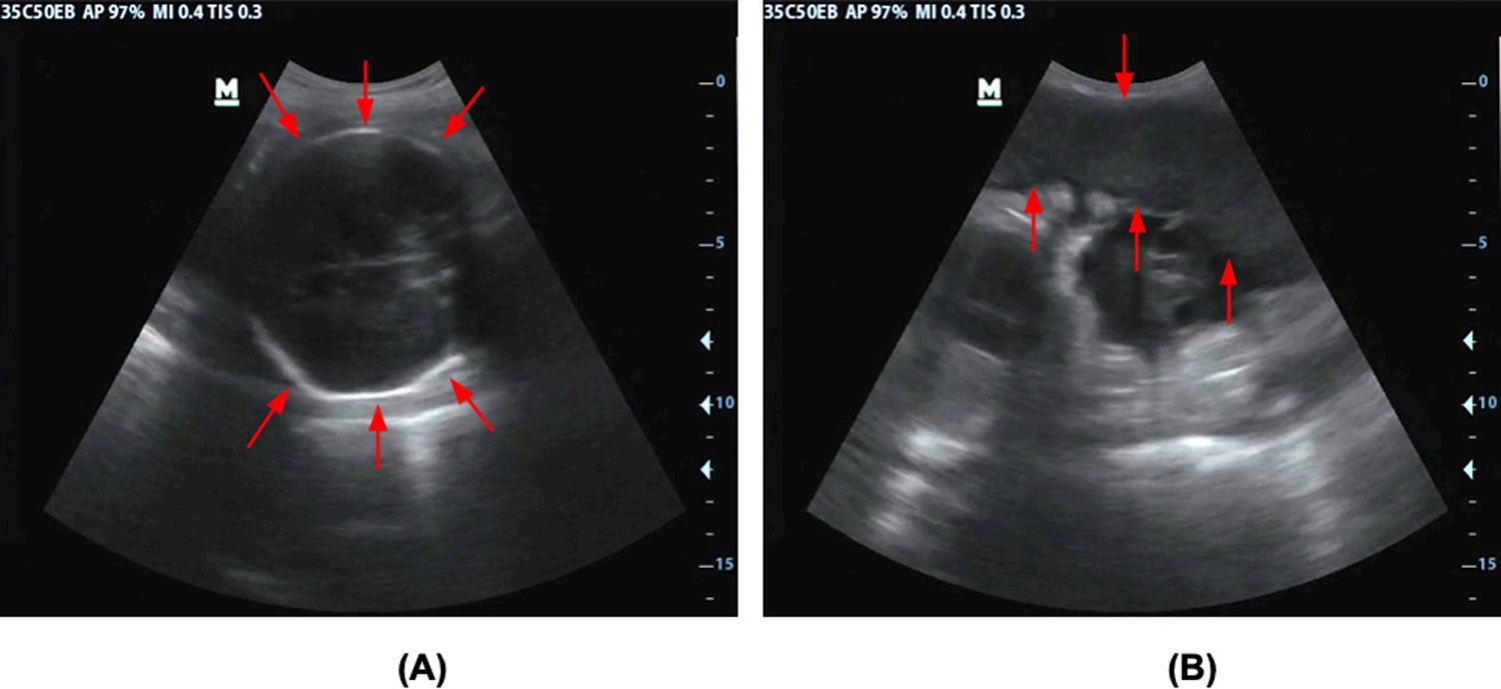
Representative 2D ultrasound images from VSI acquisition. Red arrows identify the head (A) and placenta (B).
